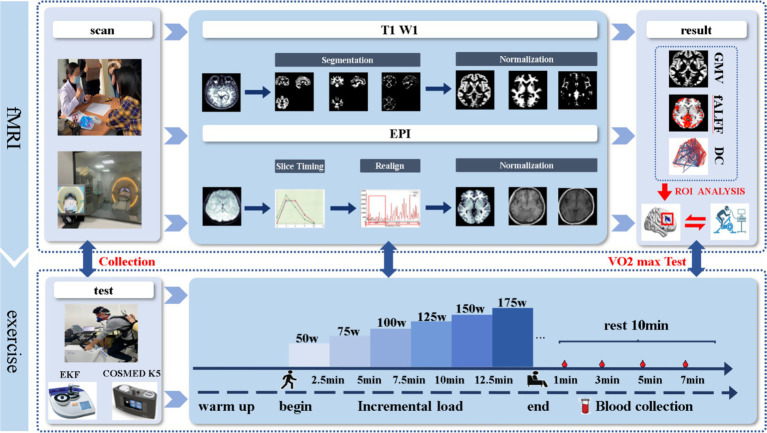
An overview of the experimental design. MRI comprised two sequences: T1-weighted structural imaging to quantify structural plasticity (GMV) and functional plasticity (fALFF and DC). During the graded incremental load test, gas exchange variables, heart rate, and earlobe capillary blood lactate were recorded.
